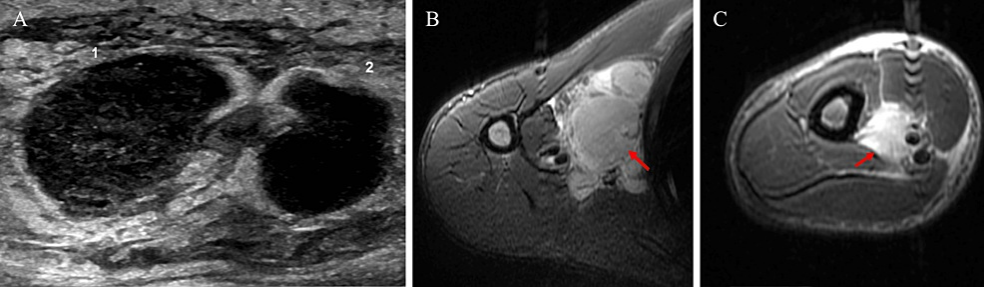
A: Ultrasound of the right distal humerus, bilobed structure measuring 3.1 x 1.2 cm, surrounding tissues are edematous; B: Magnetic resonance imaging (MRI) of the right humerus, axillary lymphadenopathy measuring 3.2 x 3.4 cm; C: MRI of the right humerus, enlarged lymph node measuring 3.0 x 2.7 cm
